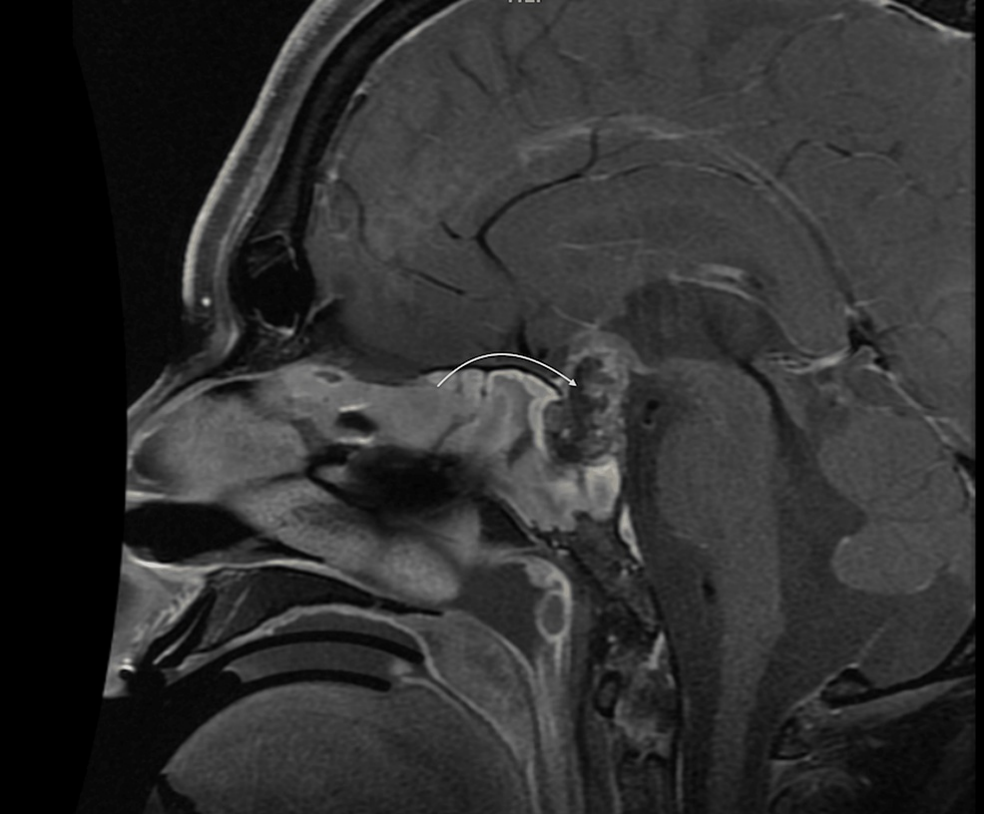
MRI brain saggital cut with contrast showing a sellar lesion with suprasellar extension (curved white arrow)
